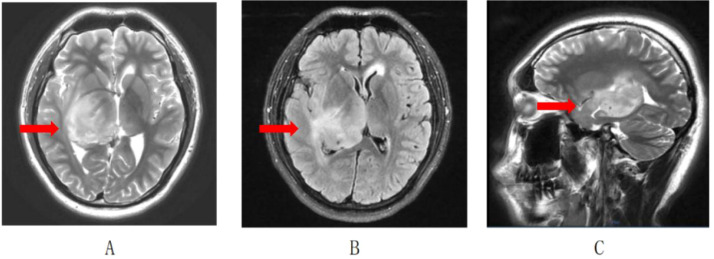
MRI revealed a large mass lesion in the right thalamus and basal ganglia. (A) T2 image; (B) T2 FLAIR image; (C) T2 sagittal view.
